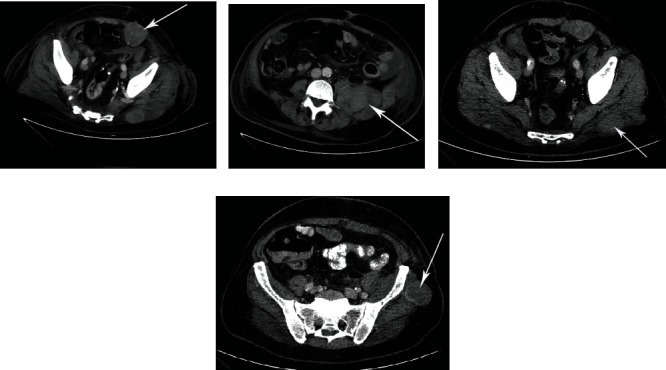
A CT scan following the surgery showed multiple residual tumors in the lower abdomen and pelvis. (a) Front abdominal wall. (b) Psoas muscle. (c) Gluteus. (d) Iliac bone.
